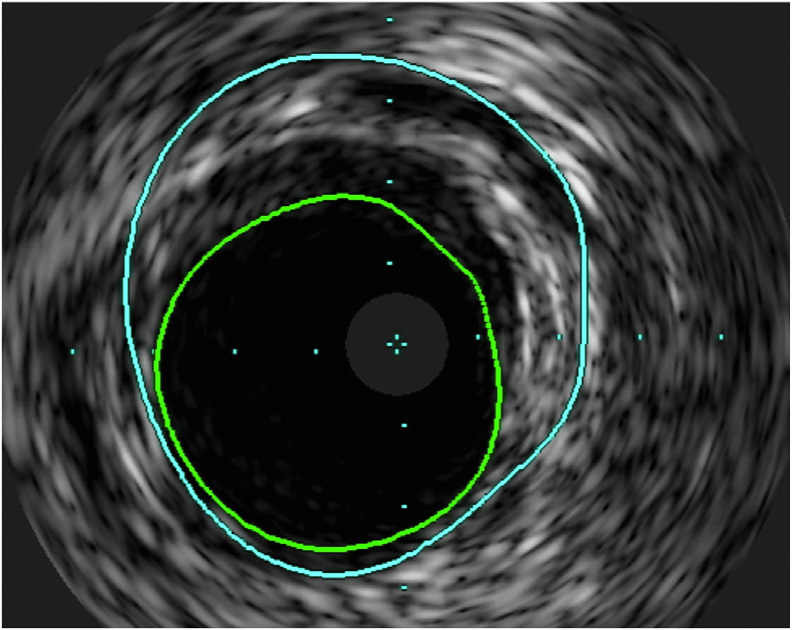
Intravascular ultrasound image showing right coronary artery dissection (green, area of coronary artery lumen; blue, area of dissection plane).
